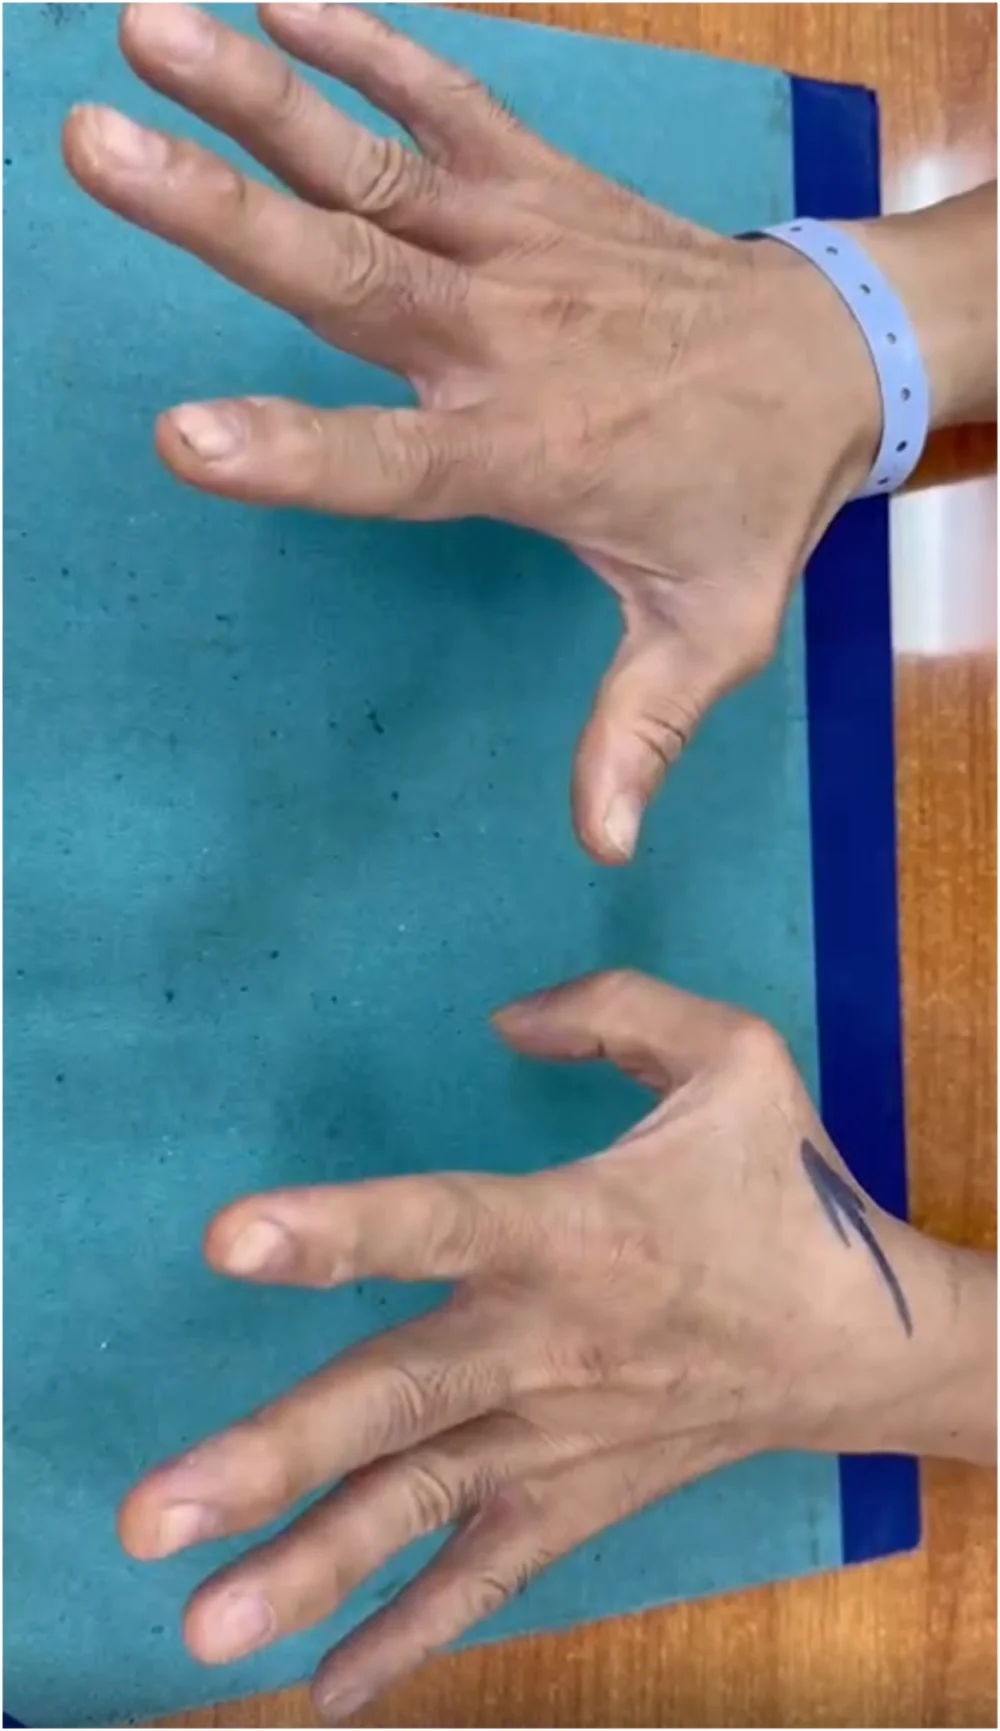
Preoperative radiographs showing minimally displaced distal radius fractures: (A) patient A (61/F); (B) patient B (41/M).
